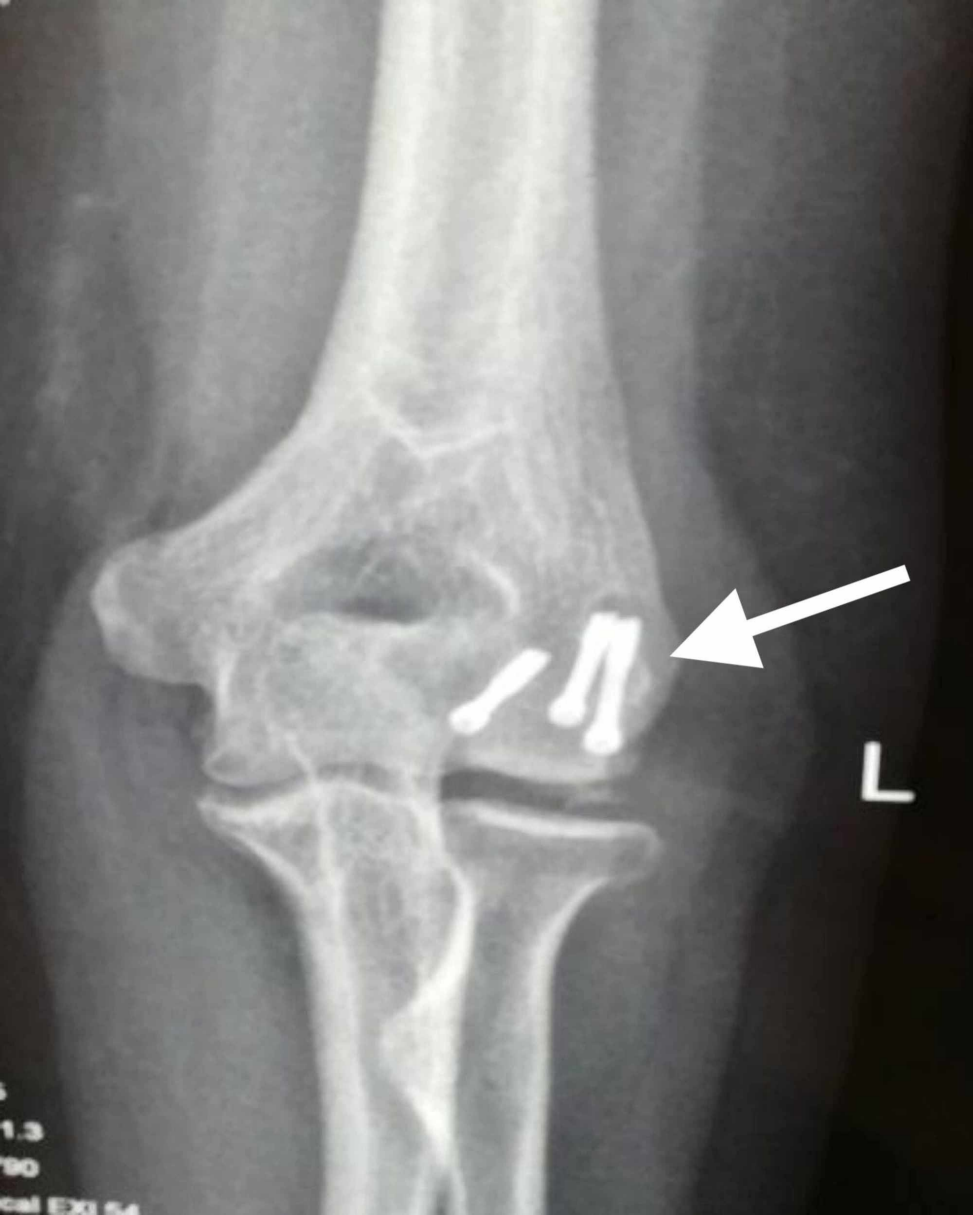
Post-operative X-ray radiograph anteroposterior(AP) view showing a fracture of the capitellum managed with Herbert screw fixation
